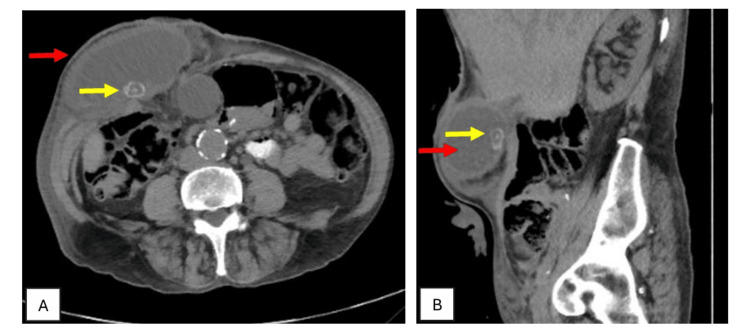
Computed tomography scan of the abdomen and pelvis showing parastomal hernia containing the gallbladder (red arrow) with cholelithiasis (yellow arrow) and cholecystitis. (A) Transverse section. (B) Sagittal section.
